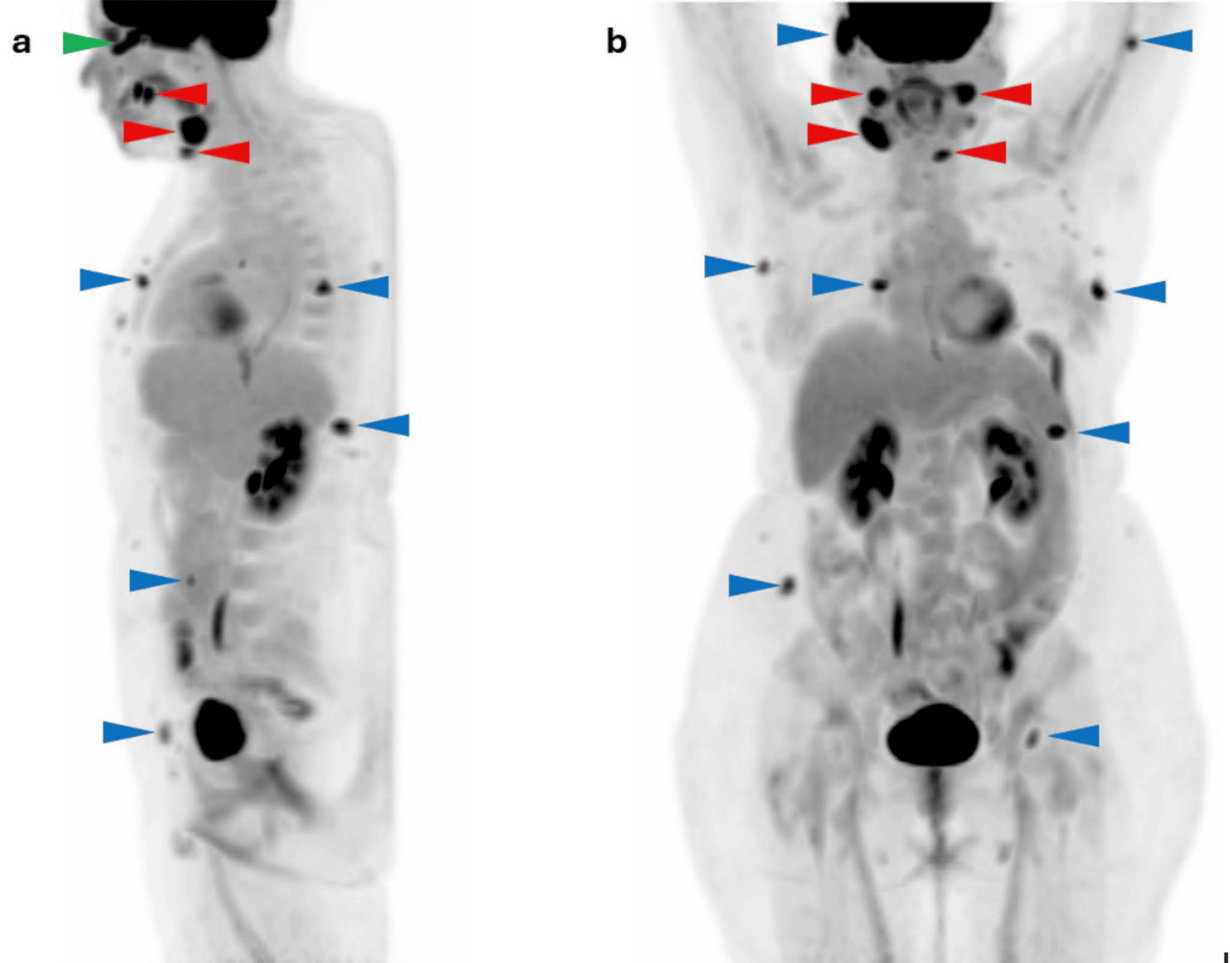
(a, b) MIP images from 18F-FDG PET/CT showing a hypermetabolic mass in the left intraconal space (green arrowhead); hypermetabolic cervical lymph nodes are seen (red arrowheads), along with multiple subcutaneous hypermetabolic foci corresponding to cutaneous lesions (blue arrowheads), consistent with disseminated lymphoproliferative disease with nodal and extranodal involvement. MIP: maximum intensity projection; 18F-FDG PET/CT: fluorine-18 fluorodeoxyglucose positron emission tomography/computed tomography.
